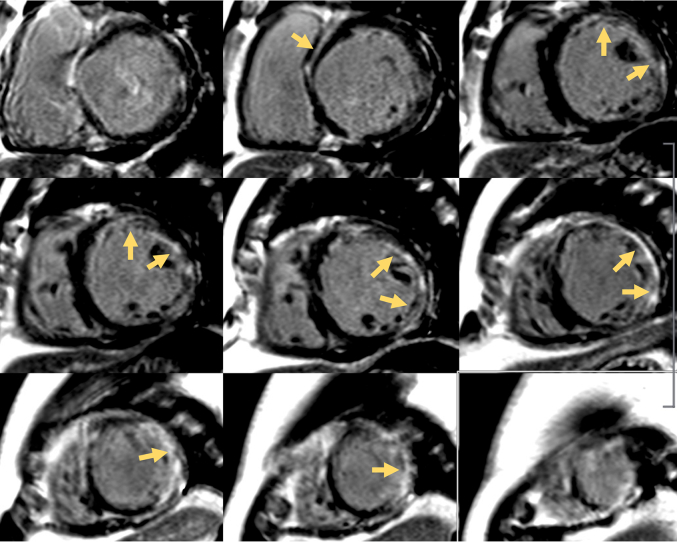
Sequential Cardiac Magnetic Resonance Imaging Findings Cardiac magnetic resonance imaging showed an extensive amount of late gadolinium enhancement involving the basal midwall septum, subendocardial basal anterior and anterolateral walls, midwall involving the mid anterolateral and inferolateral wall, and transmural apical lateral wall and apex (yellow arrows).
